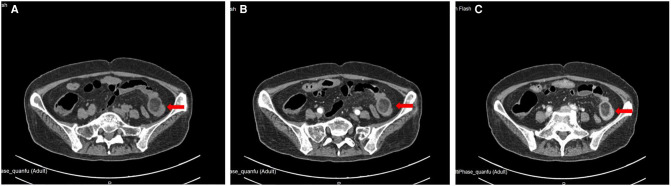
Location of small intestinal tumor in abdominal enhanced CT. (A) plain scan; (B) arterial phase; (C) venous phase.
